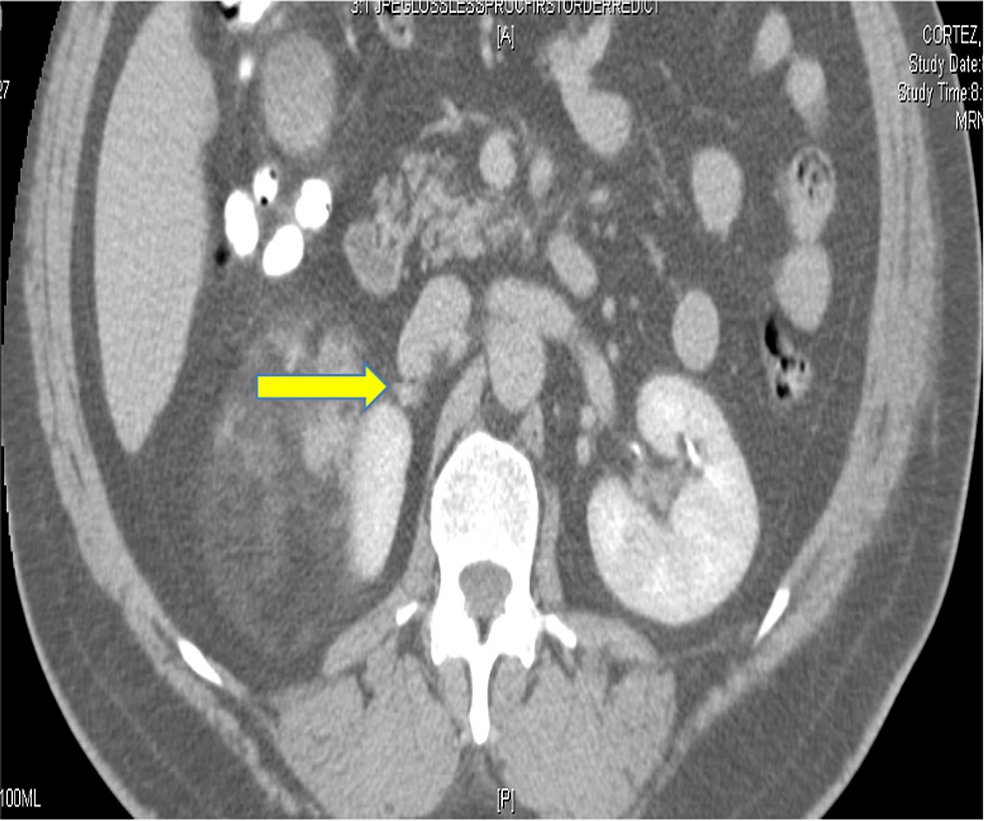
CT Portal Venous Phase - Axial View Image demonstrates a draining right adrenal vein (yellow arrow) which was ligated during surgery.
